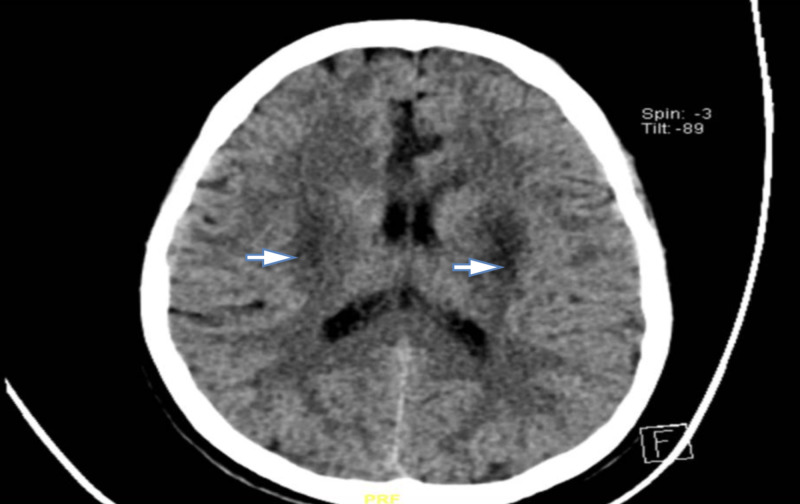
Non-enhanced CT scan of the brain showing bilateral basal ganglia hypodensities
